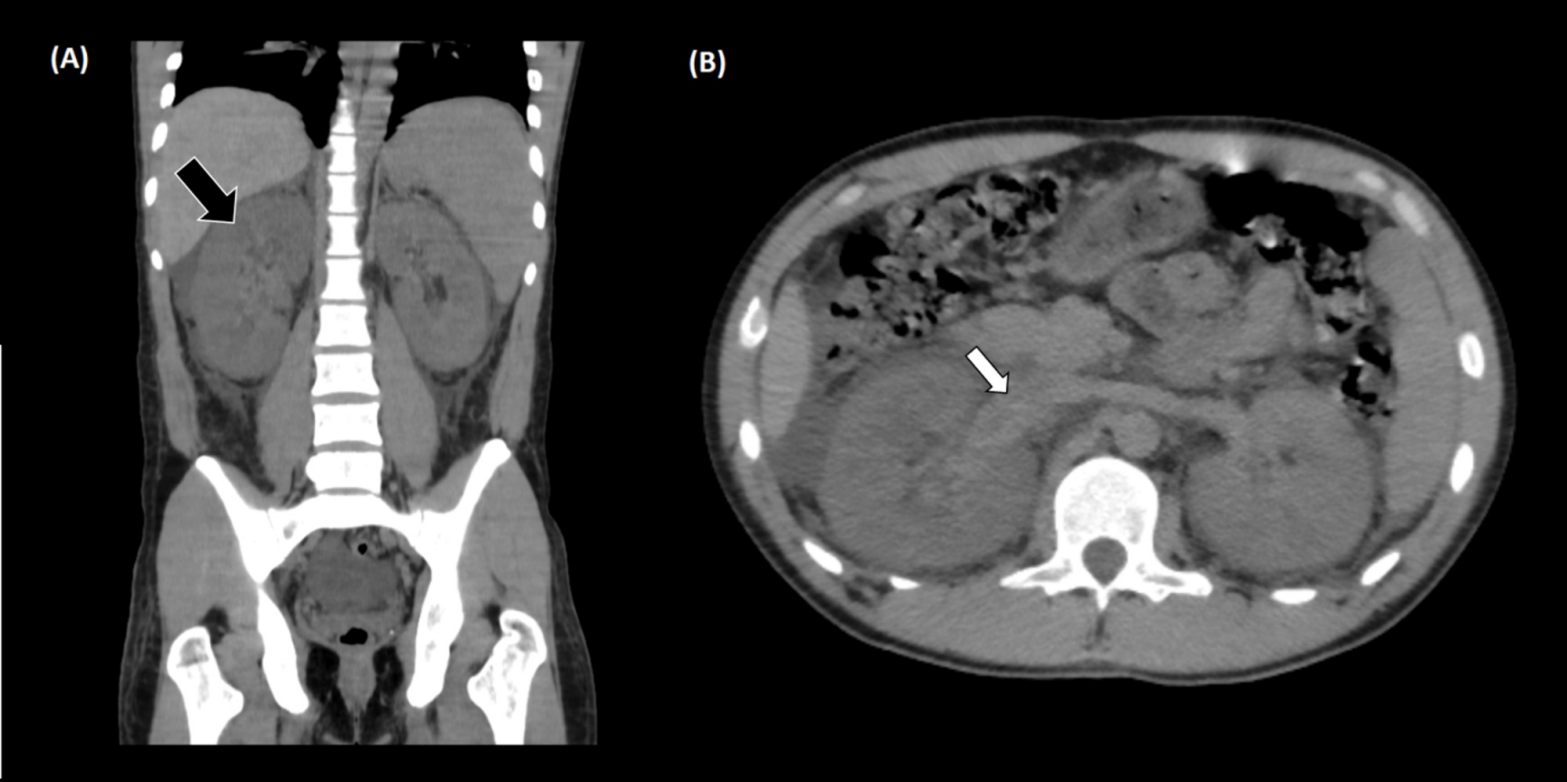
Non-contrast-enhanced CT of the abdomen Non-contrast-enhanced computed tomography of the abdomen showed an increase in the right kidney size (black arrow) and the presence of heterogeneous content in the right renal pelvis (white arrow), with no signs of obstruction due to urinary tract calculi.
